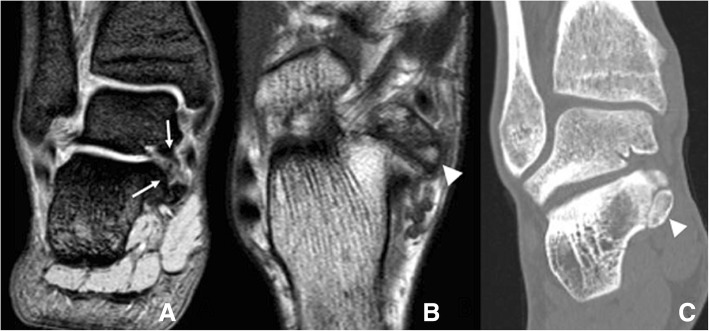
Os sustentaculi. a 17-year-old man referred with the suspicion of peroneal tenosynovitis. a Coronal T2-weighted fast field echo (FFE) demonstrates a talocalcaneal coalition (white arrows). b On axial FSE T1, a small ossicle is visible, interposed in between the two coalescent bones, in keeping with an os sustentaculi (arrowhead). c Reconstructed coronal CT image in the same patient, demonstrating the os sustentaculi (arrowhead), in close relation with the sustentaculum tali
